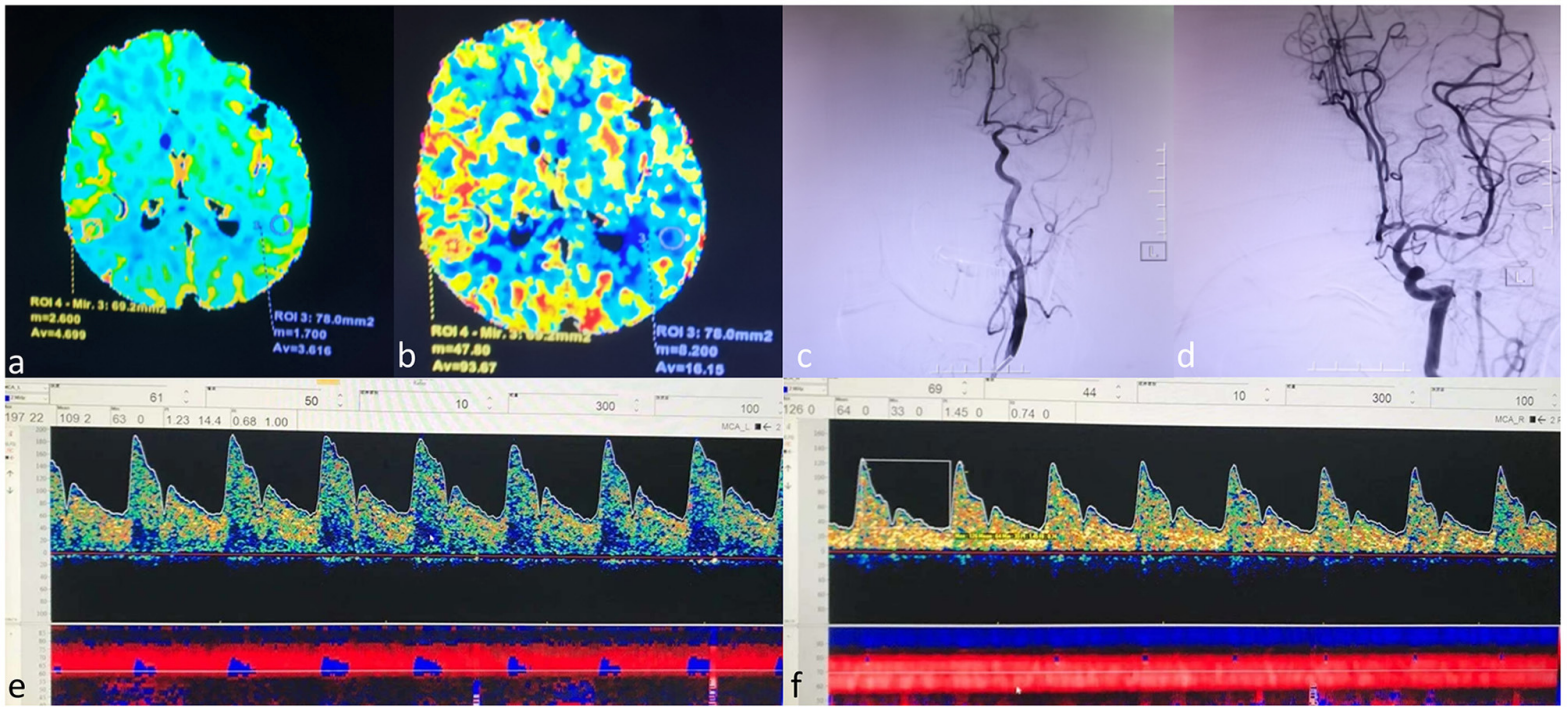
A case example. An 80-year-old female who received a successful mechanical thrombectomy in the left middle cerebral artery (MCA) branch M1. The CTP series showed that CBV did not increase (a). Mildly increased CBF (b). The left MCA occluded entirely on DSA before EVT (c). The left MCA reappeared entirely on DSA after EVT (d). The PSV of the left MCA was 197 cm/s, and MFV increased higher than 125% compared to that on the right side [(e), left MCA; (f), right MCA]. The patient was given nicardipine until the flow velocity of the left MCA was restored to the normal. The patient had no hemorrhagic transformation and achieved an mRS score of 0 at 90 days. MCA=middle cerebral artery, CTP=computed tomography perfusion, CBV=cerebral blood volume, CBF, cerebral blood flow, DSA, digital subtraction angiography, EVT, endovascular thrombectomy, PSV, peak systolic velocity, MFV, mean flow velocity.
